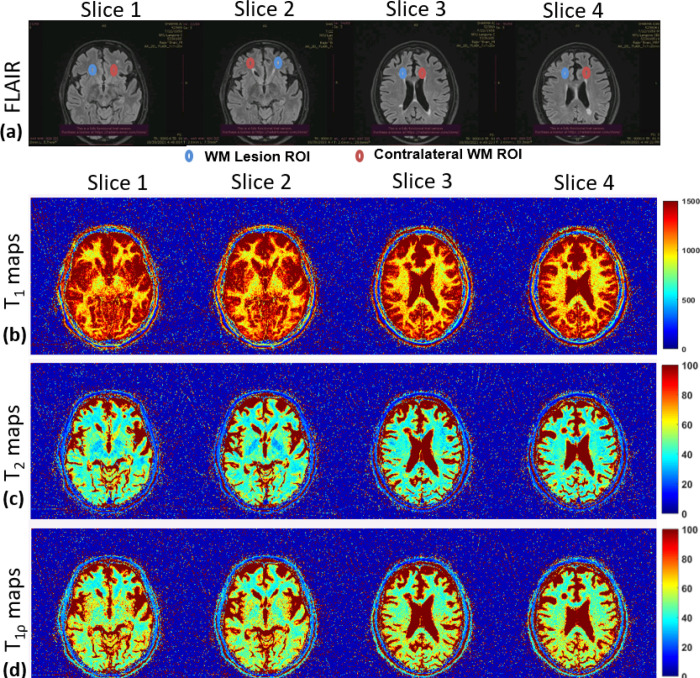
3D-MRF results in MS patient. (a) shows clinical imaging with FLAIR MRI four slices with identified lesions. Blue ROIs are drawn around the lesions, and contralateral ROIs are drawn in red. (b) to (d) show T_1_ maps, T_2_ maps and T_1ρ_ maps, respectively for each of the slices shown in (a)
